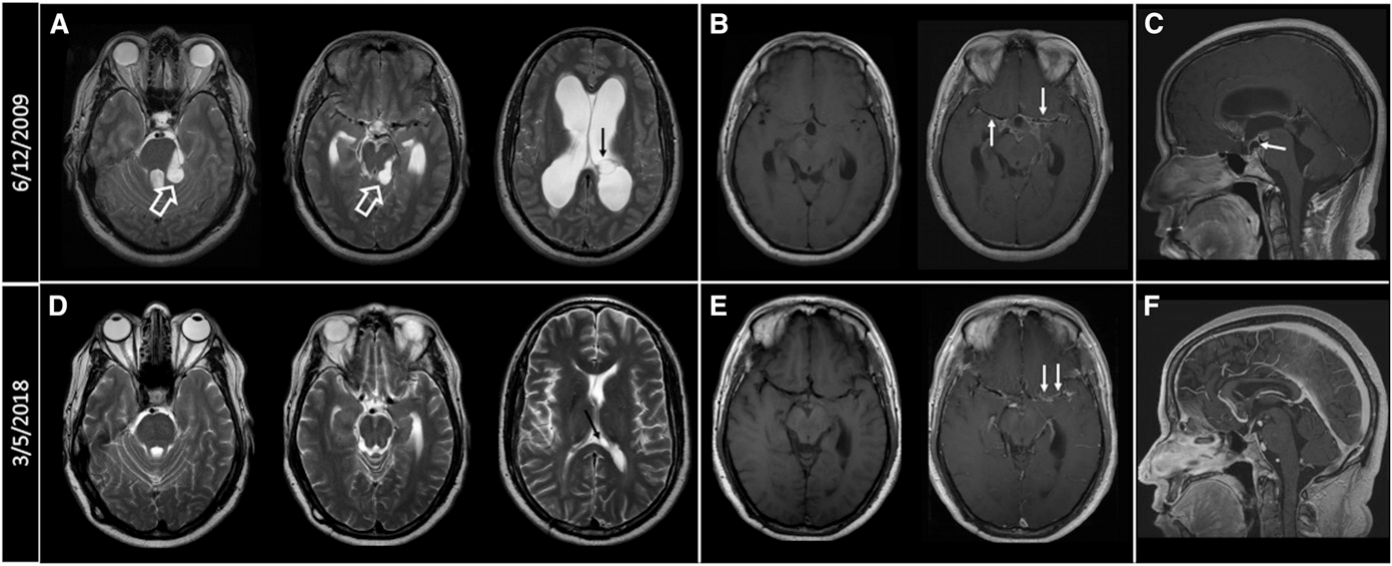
(A) Axial T2-weighted images at the levels of the pons, midbrain, and lateral ventricles. Cystic masses are seen in the perimesencephalic cistern (white hollow arrows) with secondary distortion of adjacent brain stem structures. Hydrocephalus is noted with transependymal cerebrospinal fluid seepage. A left lateral ventricular cyst can also be seen (black arrow). (B) Pre- and postcontrast T1-weighted images showing abnormal enhancement along the courses of the middle cerebral arteries (MCAs) and anterior cerebral arteries (ACAs) and extending into the interpeduncular cistern (white arrows). (C) Sagittal postcontrast T1-weighted images showing suprasellar rim enhancing cyst anteriorly displacing the pituitary stalk (white arrow). (D) Axial T2-weighted images obtained on March 5, 2018, showing resolution of cystic masses and mass effect on the brain stem structures. The ventricular cyst has markedly decreased in size (black arrow). (E) Pre- and postcontrast T1-weighted images show significant improvement of the perivascular and meningeal enhancing lesions (white arrows). (F) Sagittal postcontrast T1-weighted images show resolution of the suprasellar cyst.
